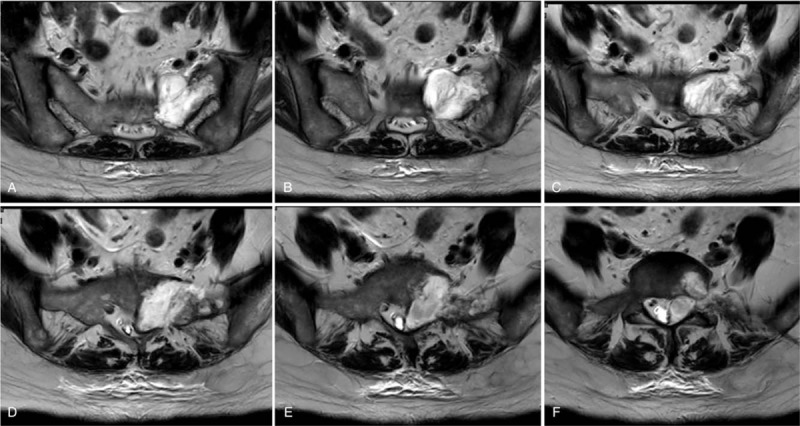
(A–F) Preoperative transverse MRI images showing sacral involvement of chondrosarcoma. Tumor infiltrated through the sacrum body into the posterior elements, resulting in severe spinal cord compression.
